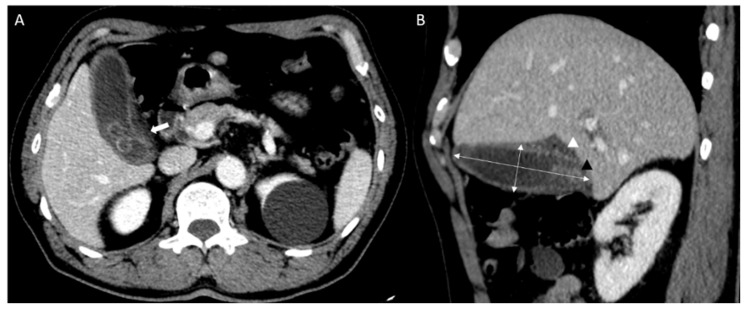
Abdominal computed tomography (CT) findings of acute cholecystitis in a 71-year-old with leukocytosis and right upper quadrant pain ((A) axial scan, (B) sagittal scan). Abdominal CT results showing gallbladder (GB) distension (double arrow; GB width, 3.36 cm and GB length, 9.34 cm) with impacted GB stone (black triangle). It demonstrates fat infiltration (white arrow) and pericholecystic fluid collection (white triangle).
